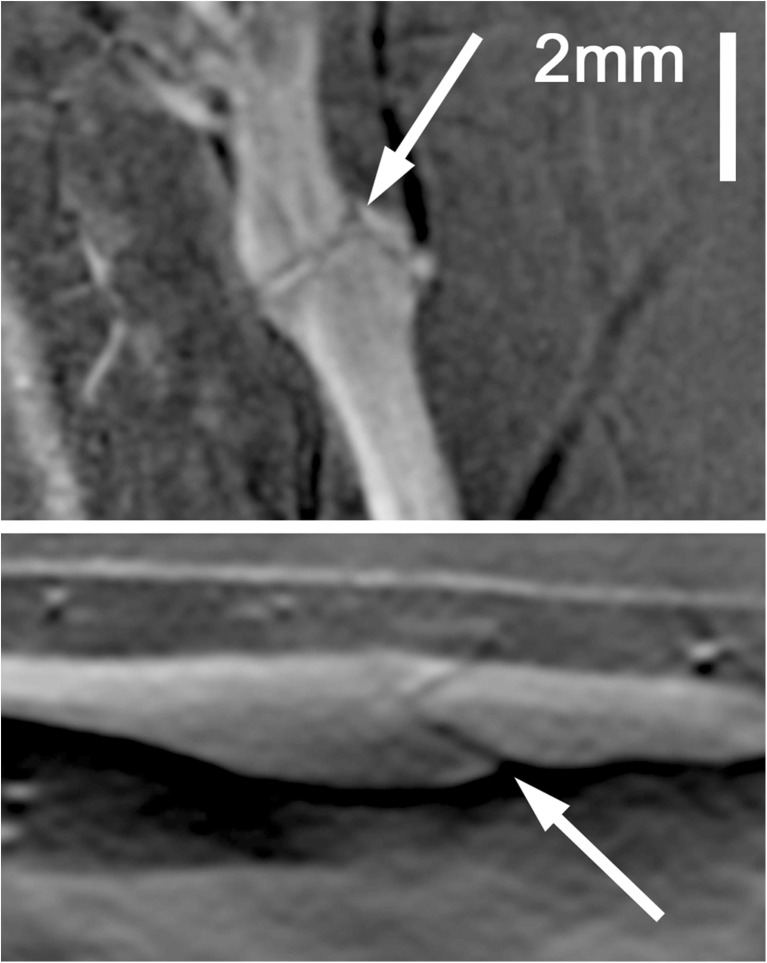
Example coronal and sagittal maximum intensity projection (MIP) images through a superficial foot vein of a healthy volunteer, depicting a pair of angled, linear webs of reduced signal (*arrows*) within the vessel at a site of slight venous dilatation. These were not visible on ultrasound, but from their morphology and location they are presumed to represent a venous valve
